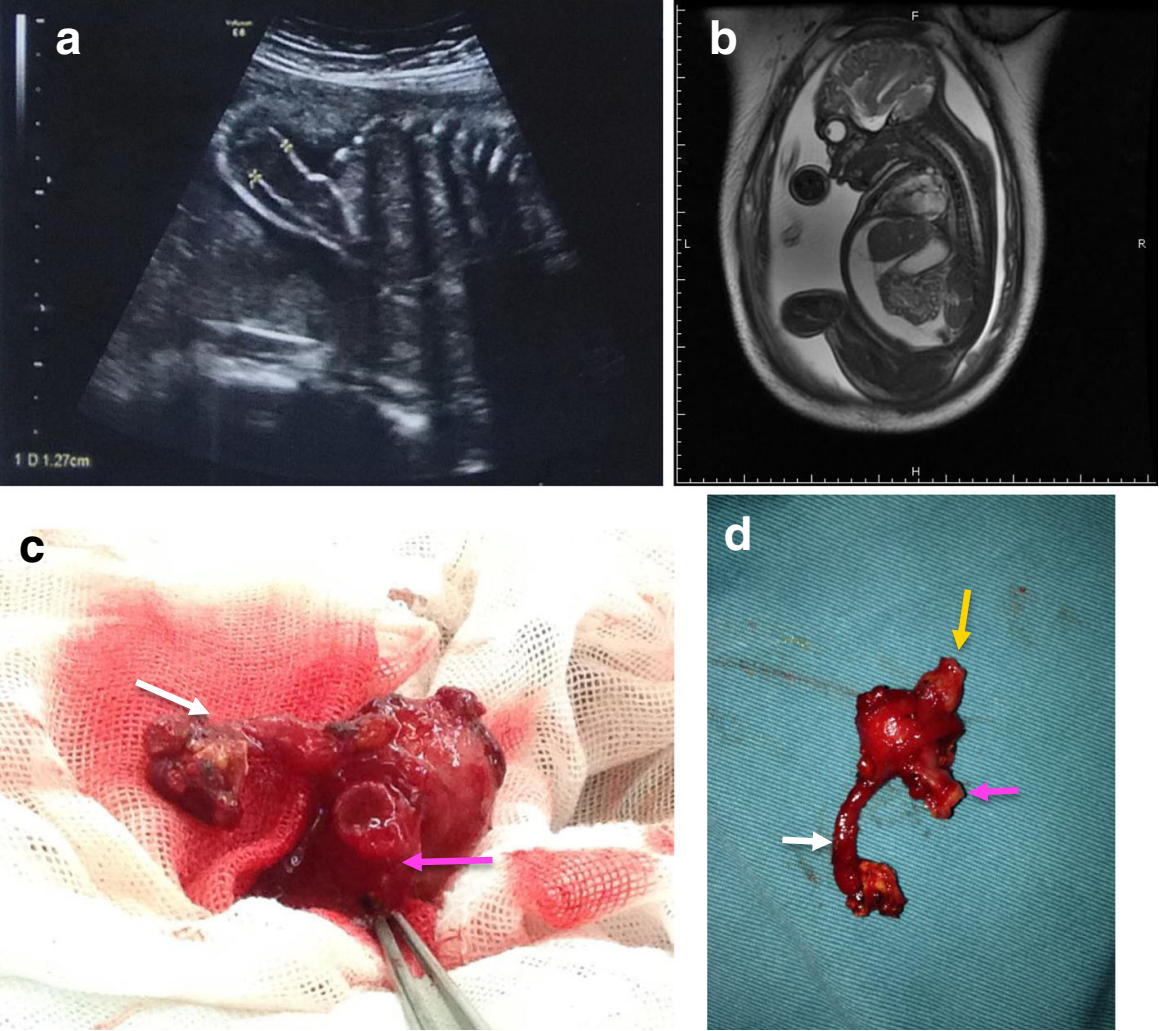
Imaging examination in fetal period and intraoperative finding for Case 1. a Prenatal US examination in 26-week gestation revealed the fetal intestine was dilated obviously as the inner diameter to 12.7 mm. b Sagittal MRI scanning in 34-week gestation showed the ascites had been increasing constantly with 32 mm in depth. c, d Gangrenous appendicitis with perforation was near the tip as well as a perforated lesion at the terminal ileum. We performed a limited ileocaecal resection with ileo-ascending colon anastomosis (white arrow: the gangrenous appendix, pink arrow: terminal ileum, yellow arrow: ascending colon)
